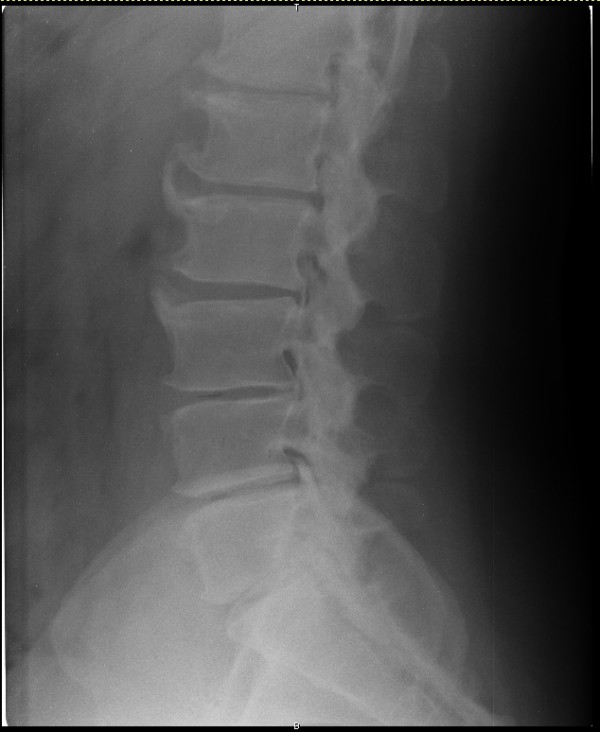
Representative radiograph of anterior lumbar vertebral osteophytes; 72 years old male.
